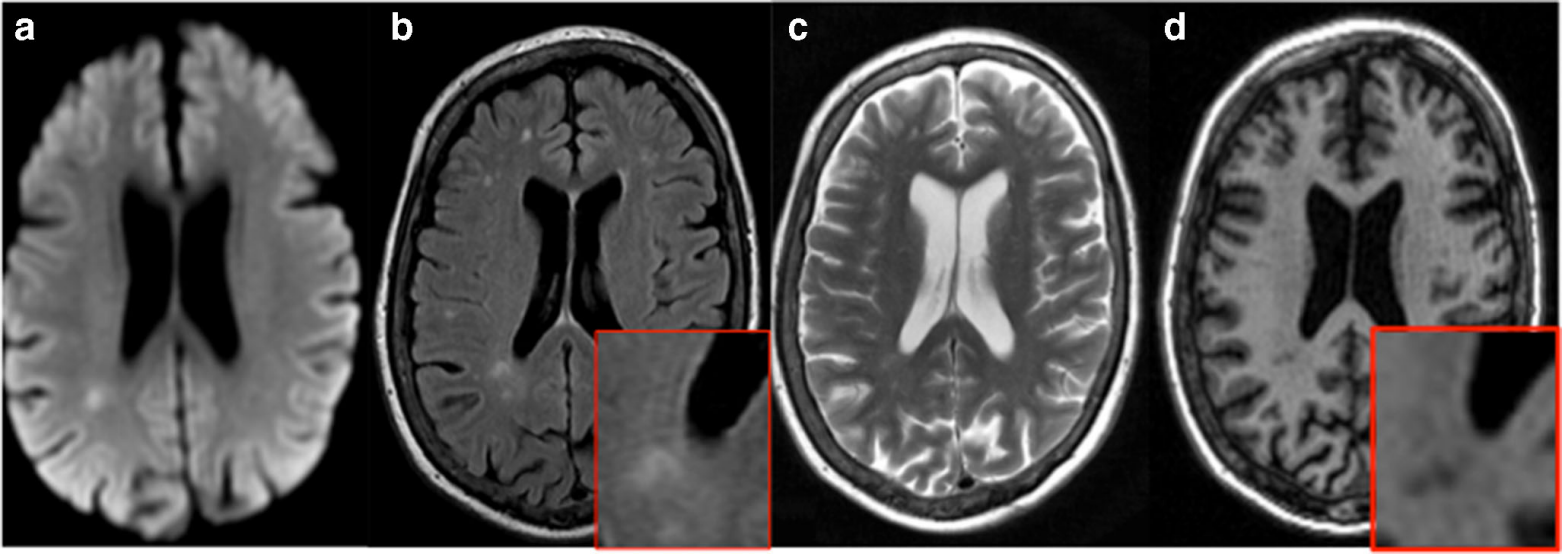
Example of an RSSI with subtle cavitation at 1 year. Index RSSI on DWI MRI (A). At 1-year post-stroke, there is no indication for cavitation on FLAIR- or T2-weighted scans (B and C). Also, T1 shows only subtle cavity formation, which becomes clearer after image magnification (D)
